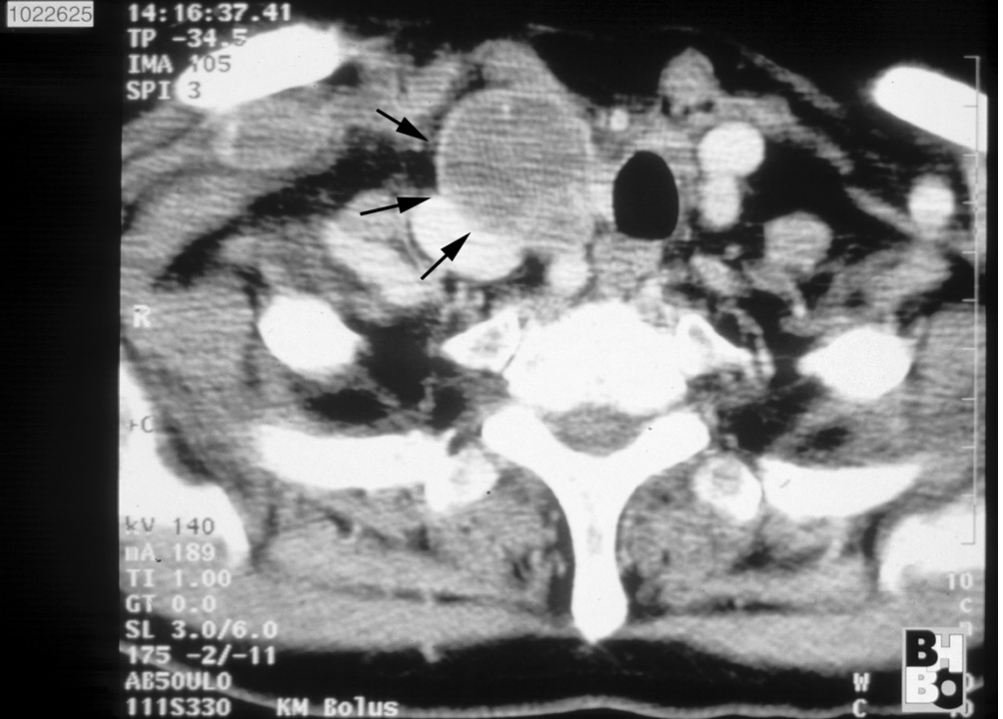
CT scan of the neck and upper medastinum: Confirmation of a soft tissue tumour (→) 4 cm in size. Expansive tumour growth displaced the trachea to the left and compressed the adjacent vessels.
